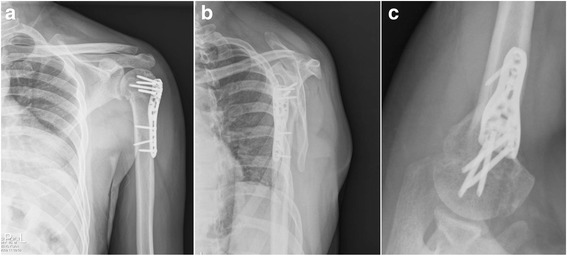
Postoperative radiographs at three months post-surgery, showing osseous union. a Anteroposterior projection. b Lateral projection. c Axial projection
